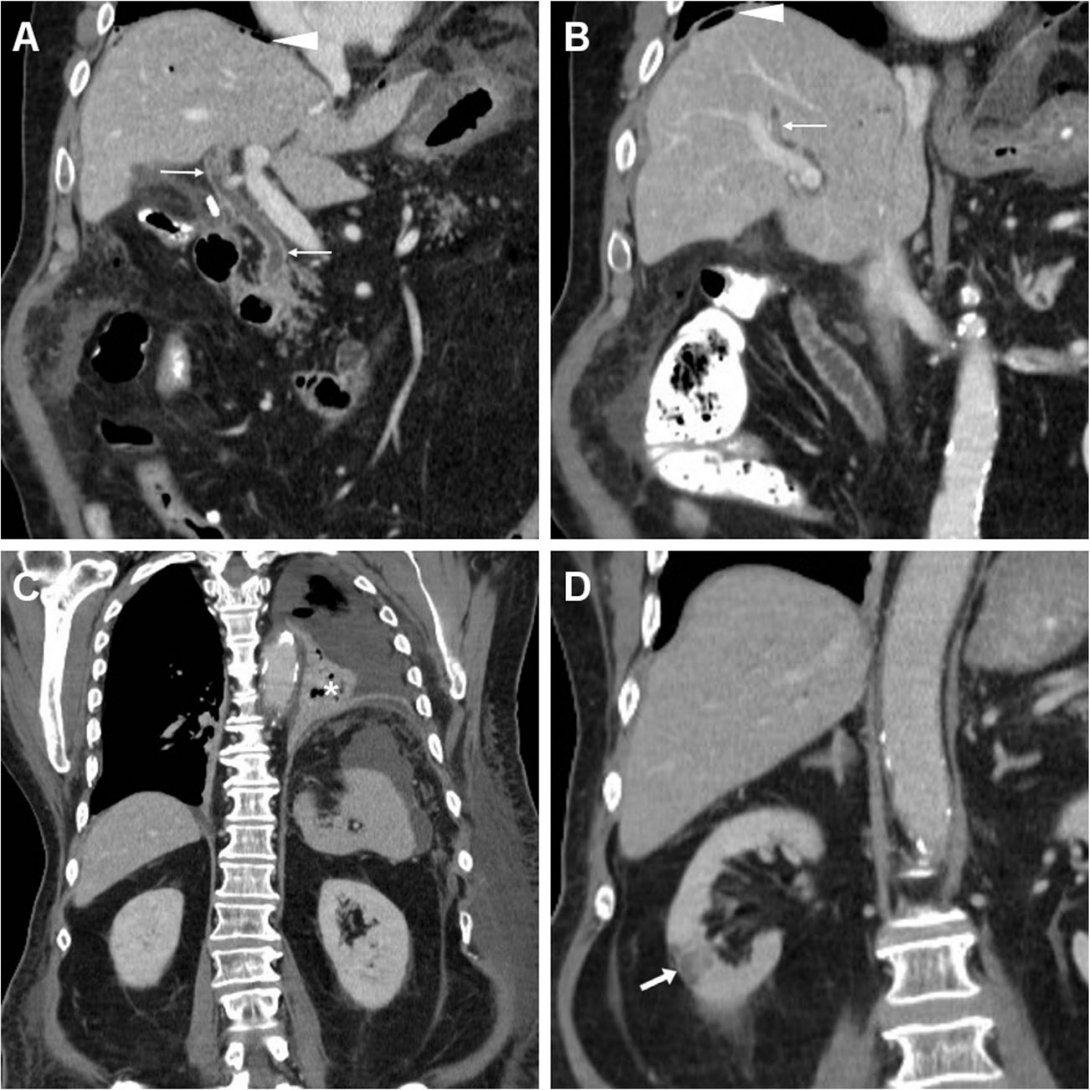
Cholangitis and sepsis in an 81-year-old female following endoscopic stone removal using a Fogarty catheter. Multiplanar contrast-enhanced CT images showed minimal pneumoperitoneum (*arrowheads*) and peritoneal effusion, thickened enhancing walls of CBD and main intrahepatic ducts (*thin arrows*). Additionally, left-sided pneumonia (* in c) and a limited renal infarct (*arrow* in d) were seen. Worsening clinical conditions dictated surgery, which revealed biliary peritonitis from contained cystic duct injury. The patient recovered after a long intensive care unit stay
